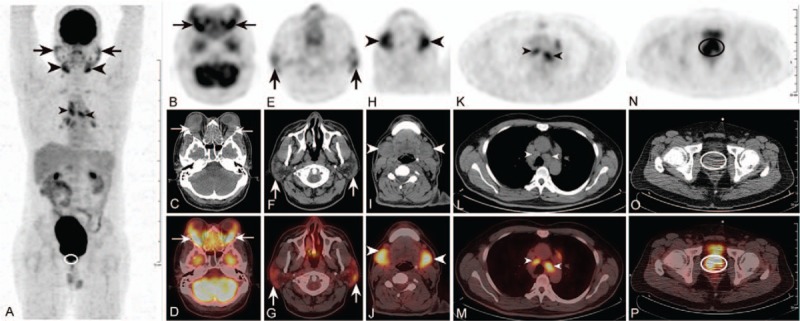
(A) The MIP image shows multiple ^18^F-FDG-avid structures. (B–P) Figures displayed from top to bottom represent axial ^18^F-FDG PET, CT, and PET/CT fusion. Whole-body ^18^F-FDG PET/CT shows increased FDG uptake in the soft-tissue masses located in the bilateral orbits (SUV_max_ of 8.21; B, C, D: thin arrows), salivary glands (SUV_max_ of 4.53; A, E, F, G: thick arrows), submandibular glands (SUV_max_ of 6.56; A, H, I, J: thick arrowheads), lymph nodes (SUV_max_ of 6.21; A, K, L, M: thin arrowheads), and prostate (SUV_max_ of 7.43; A, N, O, P: hollow ellipse), suggestive of IgG4-RD involvement in the aforementioned FDG-avid structures. MIP = maximum intensity projection; ^18^F-FDG = ^18^F-fluoro-2-deoxy-D-glucose; PET/CT = positron emission tomography/computed tomography; SUV_max_ = maximal standardized uptake value; IgG4-RD = IgG4-related disease.
